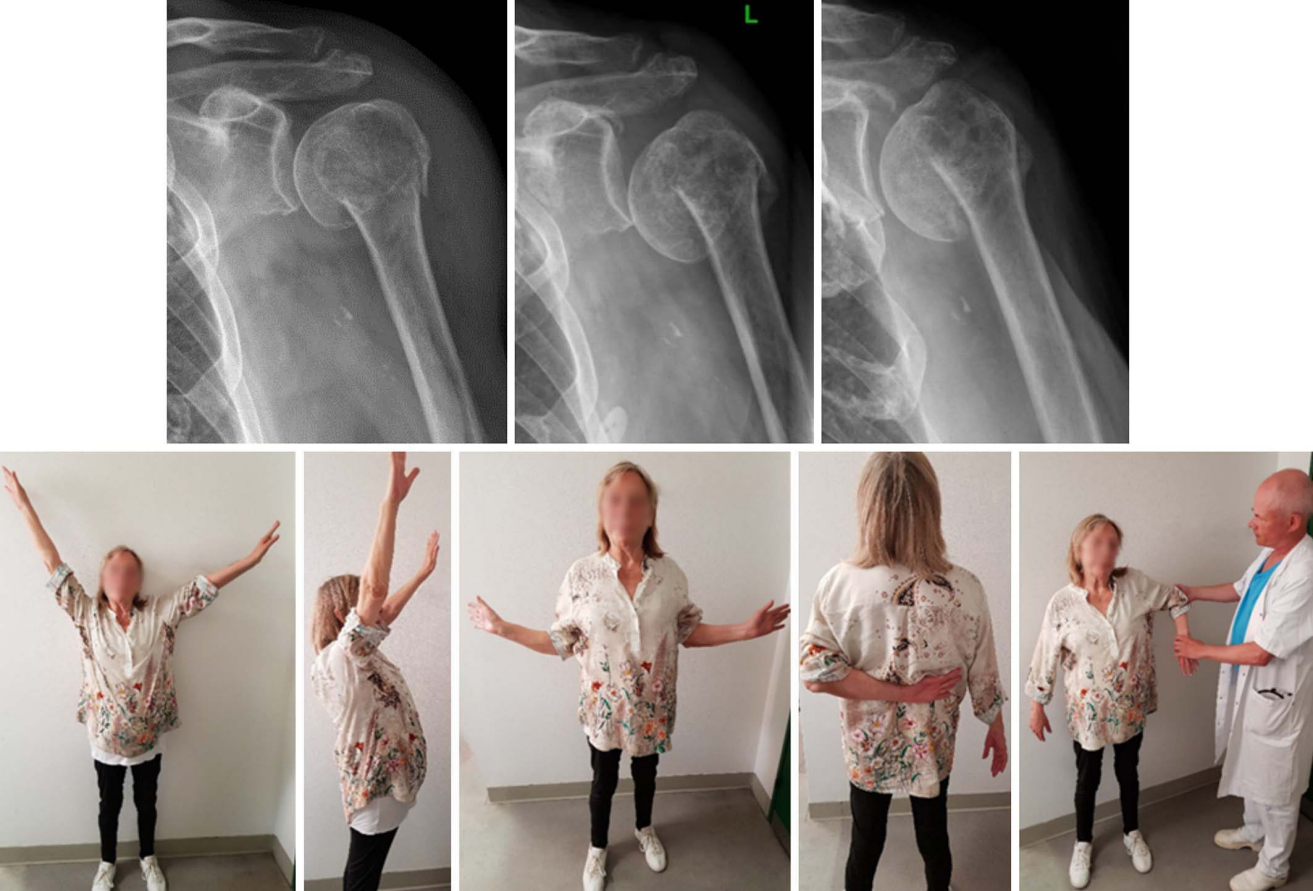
A 71-year-old female with a 2-part surgical neck fracture. The fracture collapsed into varus within the first week but eventually healed resulting in pain-free shoulder function. Radiographs at admission, 1 week, and 12 weeks. Clinical photos at 12 weeks.
